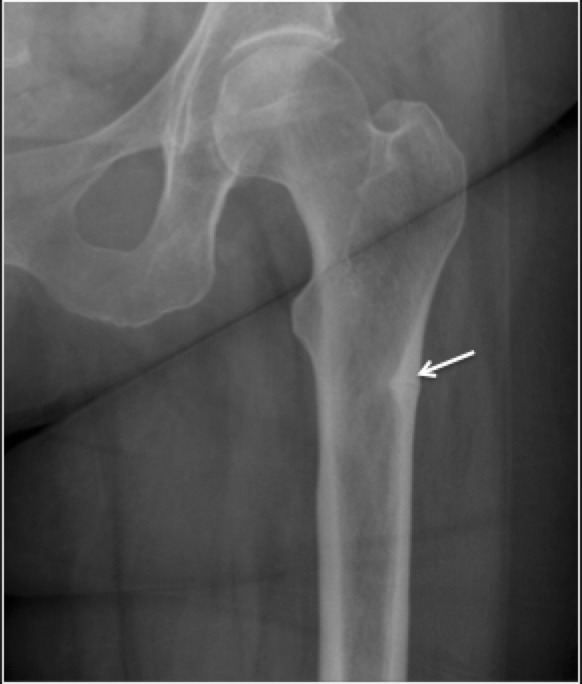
Anteroposterior radiograph of the proximal femur with atypical femoral fracture in the left proximal femur. Note the lucent transverse fracture line with endosteal and periosteal beaking (arrow).
